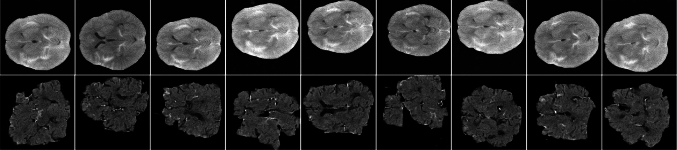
Generation of SAH CT images from pure noise. The top row shows homogeneous samples generated using DDPM with Gaussian noise. The bottom row displays mode collapse and generation failures from AnoDDPM with Simplex noise
